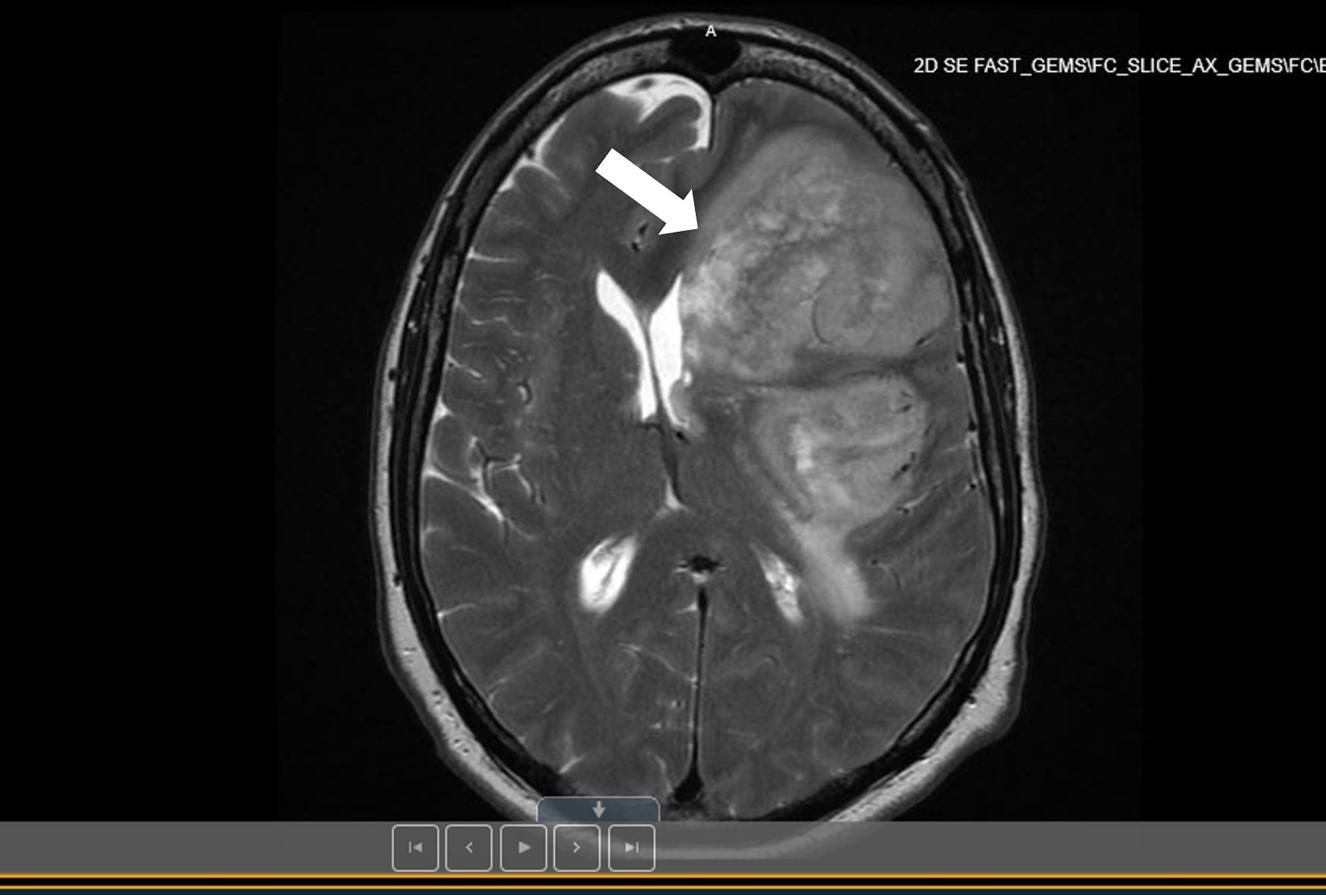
MRI of the brain reveals a large 9.5 cm infiltrating intra-axial mass in the left frontal and temporal lobe with 13 mm midline shift from left to right (indicated with a white arrow).
